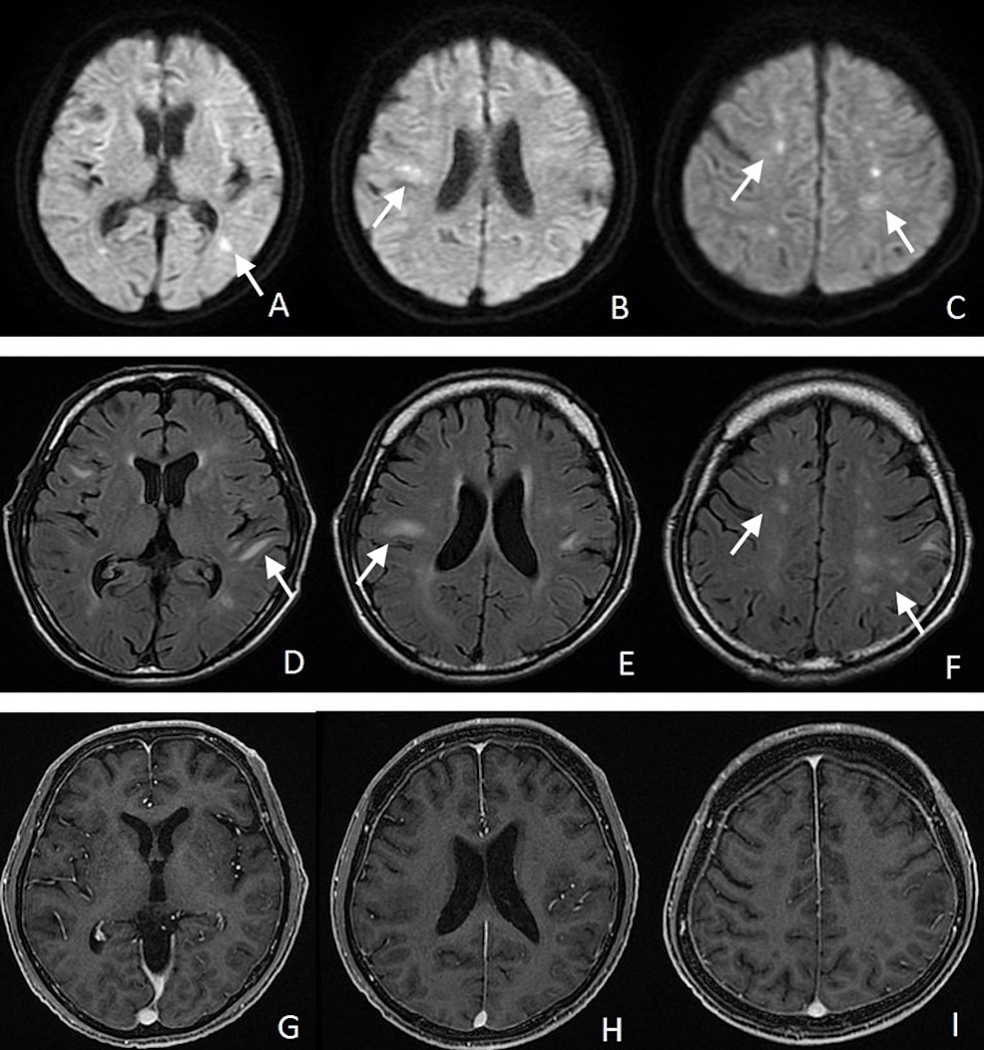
Magnetic resonance images on arrival Magnetic resonance images show the multiple lesions in the bilateral cerebral hemispheres as hyperintensity on the diffusion-weighted (A-C) and fluid-attenuated inversion recovery images (D-F). The lesions did not show any enhancements on contrast-enhanced T1-weighted images (G-I).
